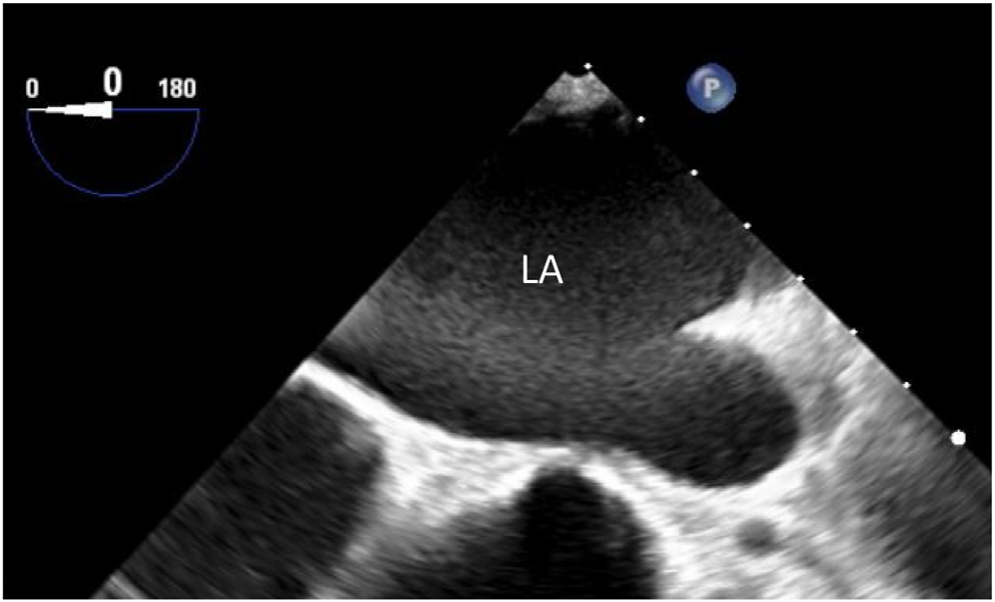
Transesophageal echocardiography performed 9 days before catheter ablation for atrial fibrillation. Spontaneous echocardiographic contrast was documented. LA = left atrium.
